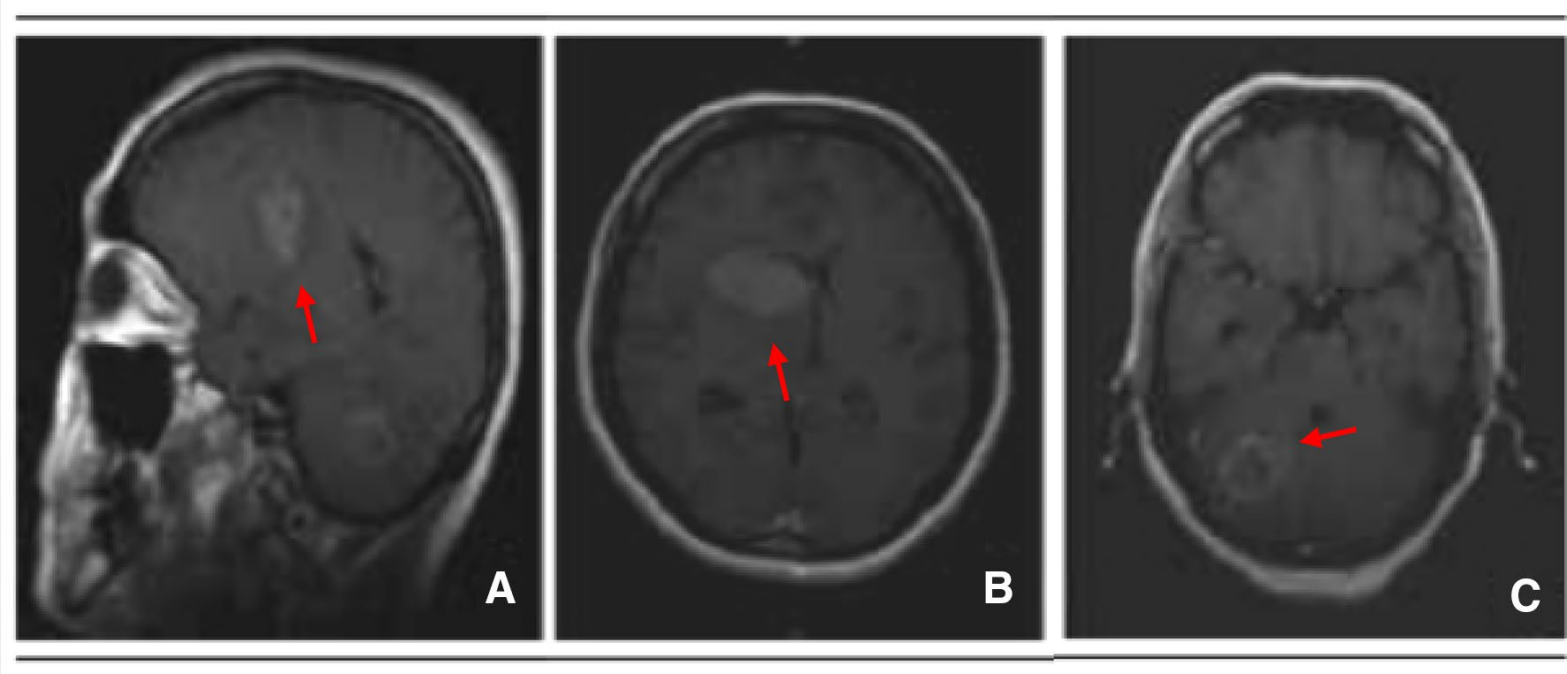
Pre-operative MRI scans exhibiting two expansive bulky lesions arising in the left thalamus (a and b) and cerebella hemisphere c with an irregular ring contrast enhancement
